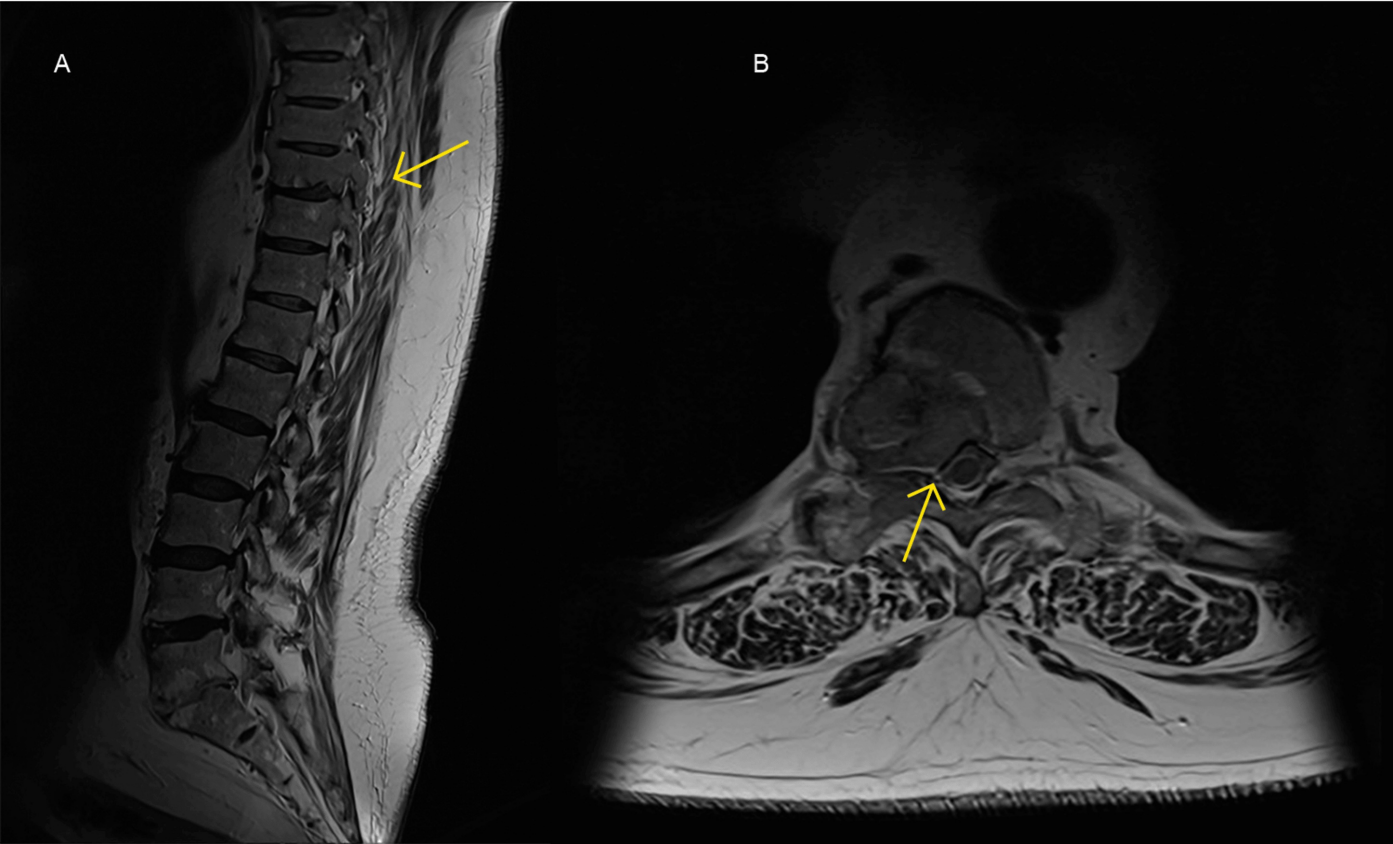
T2-weighted MRI of the thoracolumbar spine. (A) Sagittal T2 slice demonstrating multiple spinal lytic lesions with destruction of the T9 vertebra (yellow arrow); (B) Axial T2 slice at the level of T9, demonstrating expansion of the T9 vertebral body resulting in flattening of thecal sac (yellow arrow).
